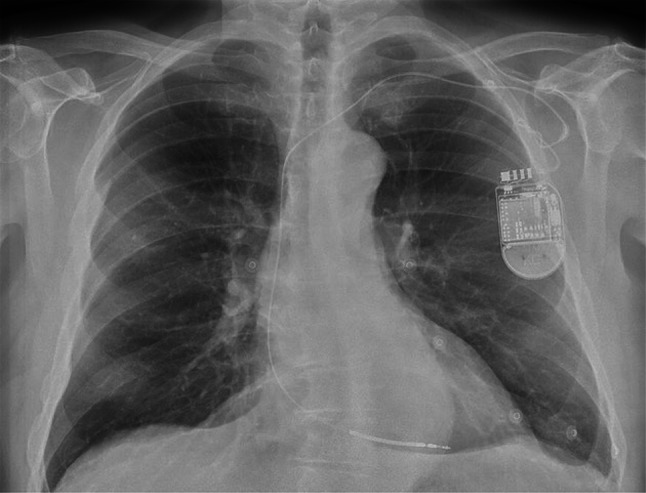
Chest radiograph taken at the emergency department
